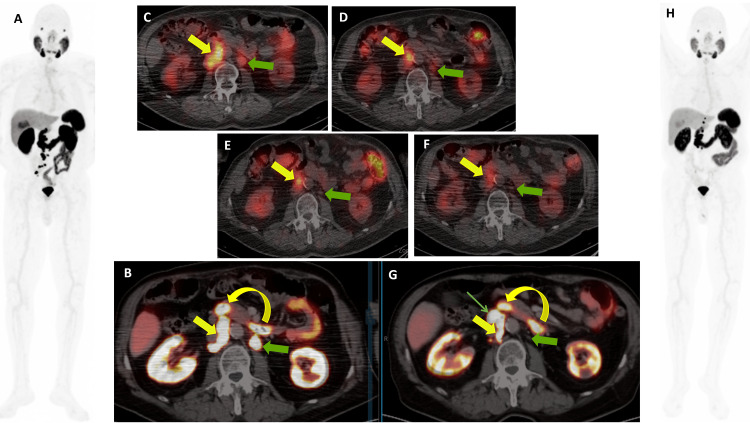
Follow-up prostate-specific membrane antigen (PSMA) PET and single-photon emission computed tomography (SPECT) imaging Follow-up Illuccix (Ga‑68 PSMA‑11) PET/CT (A, B) after two cycles of LuPSMA, IMRT to the ICC, and SBRT to pelvic nodes showed new retroperitoneal (solid yellow arrows) and pelvic lymph node metastases (the curved yellow arrow represents physiological intestinal uptake). SPECT/CT after the third, fouth, fifth, and sixth cycles (C–F) showed marked response, with decreased size and PSMA uptake in retroperitoneal nodes (solid yellow arrows) and complete regression of the left para‑aortic node (solid green arrow). PET/CT 10 weeks after the sixth cycle (G, H) showed mixed response: regression of many lymph nodes but new metastases in the precaval (outlined green arrow) and upper retroperitoneal regions. Decreased PSMA uptake in the ICC (A, H – MIP images) was also seen.
